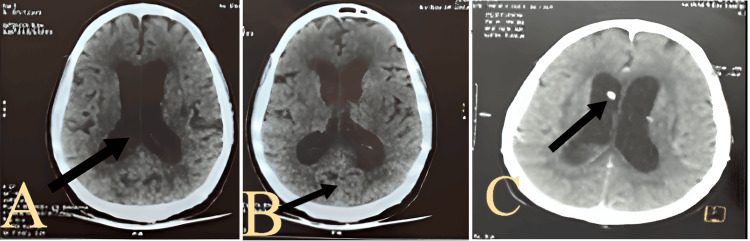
Axial non-contrast cranial CT scan showing moderate triventricular hydrocephalus (A, arrow), left cerebellar and vermian hemorrhagic stroke (B, arrow), and a drain in place (C, arrow). These images support the diagnosis of the patient described in the case presentation. The patient subsequently required placement of an EVD for CSF diversion. EVD: external ventricular drain; CT: computed tomography; CSF: cerebrospinal fluid
